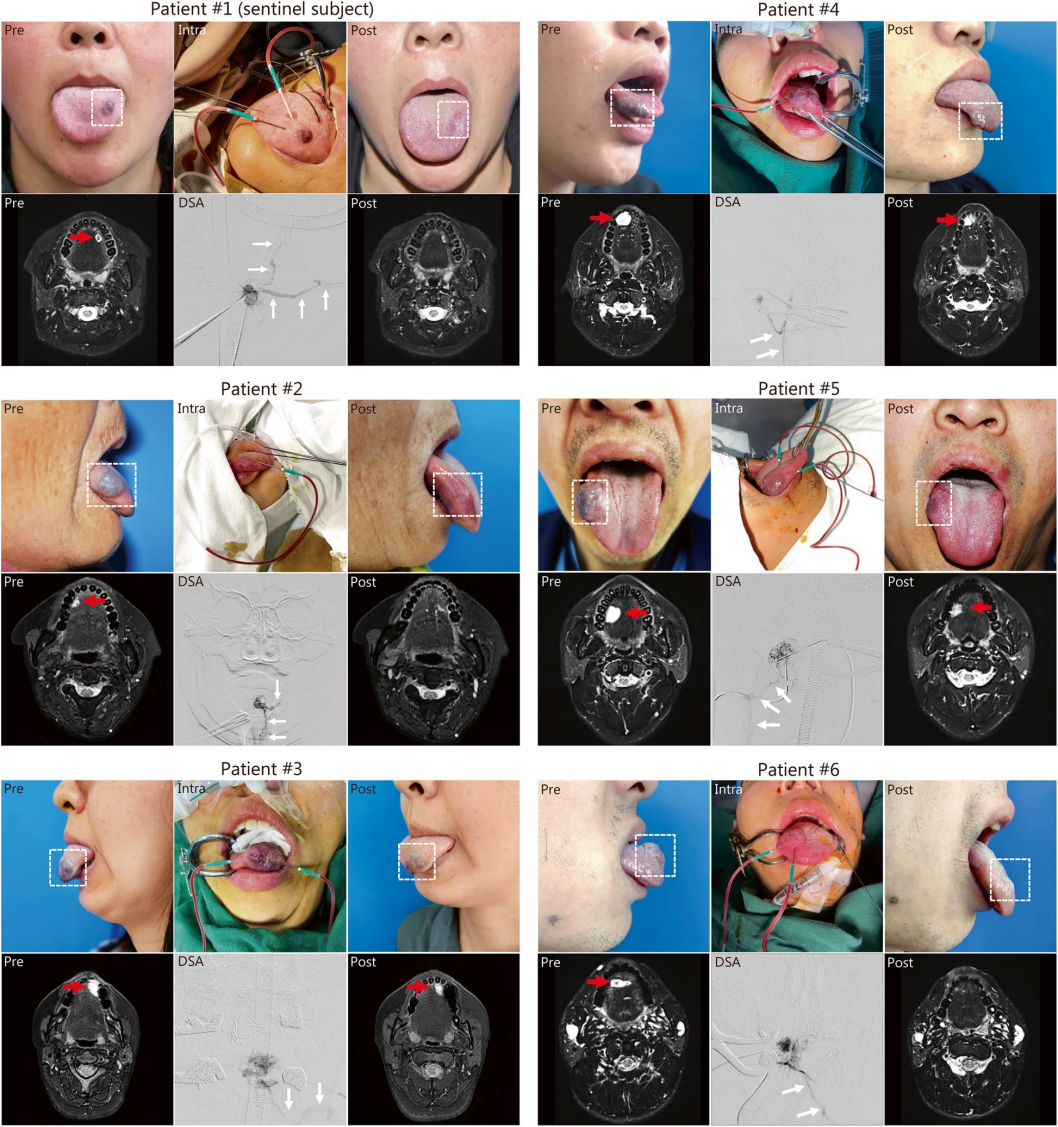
Sclerosis of patients with lingual venous malformations (VMs) by radiopaque ethanol injection (REI). Preoperative (Pre), intraoperative (Intra), and postoperative (Post) imaging and examinations of patient #1 (sentinel subject) and patients #2–6. Dotted rectangles indicate lesions of VMs; red arrows indicate VMs on magnetic resonance imaging; white arrows indicate outflowing veins of VMs. DSA digital subtraction angiography
